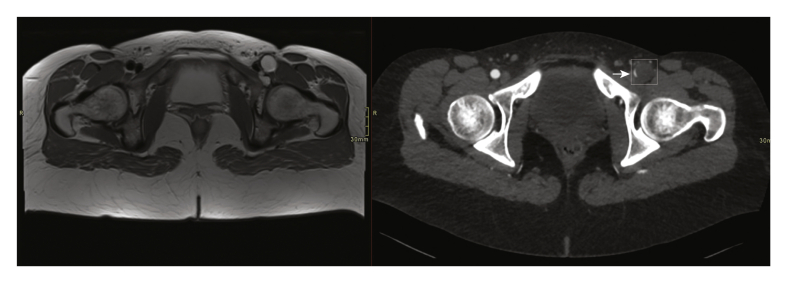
Images of the adventitial cyst at the level of the common femoral artery on the left (T2 weighted MRI). The image on the right shows a post-contrast CT angiogram with a slit like lumen of the common femoral artery (arrow) and a normal lumen on the right side of it. The hypodense dilated aspect of the common femoral artery on the left (box) is not a thrombosed dissection or aneurysm as we see on the T2 weighted MRI image that shows a bright (water) signal in the cystic structure.
